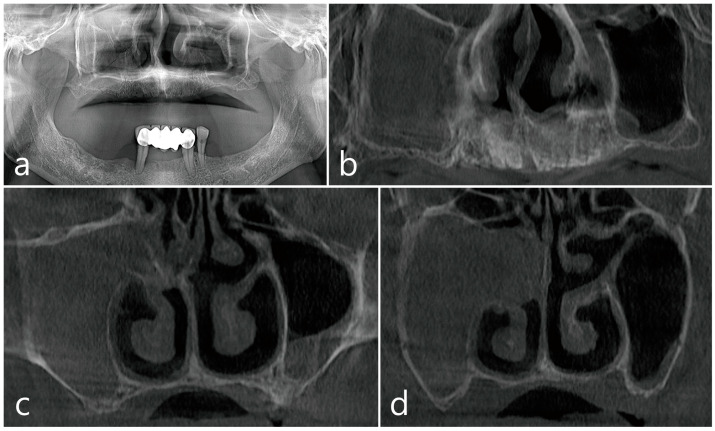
Case 1. Preoperative radiographs. (a) Severe atrophy of the residual alveolar bone shown in the panoramic image. (b) Panoramic image of CBCT scan showing complete opacification in the unilateral right maxillary sinus. (c,d) Coronal images of CBCT showing the blockage of natural ostium and severely thickened mucosa occupying up to the middle meatus. CBCT; cone beam computed tomography.
